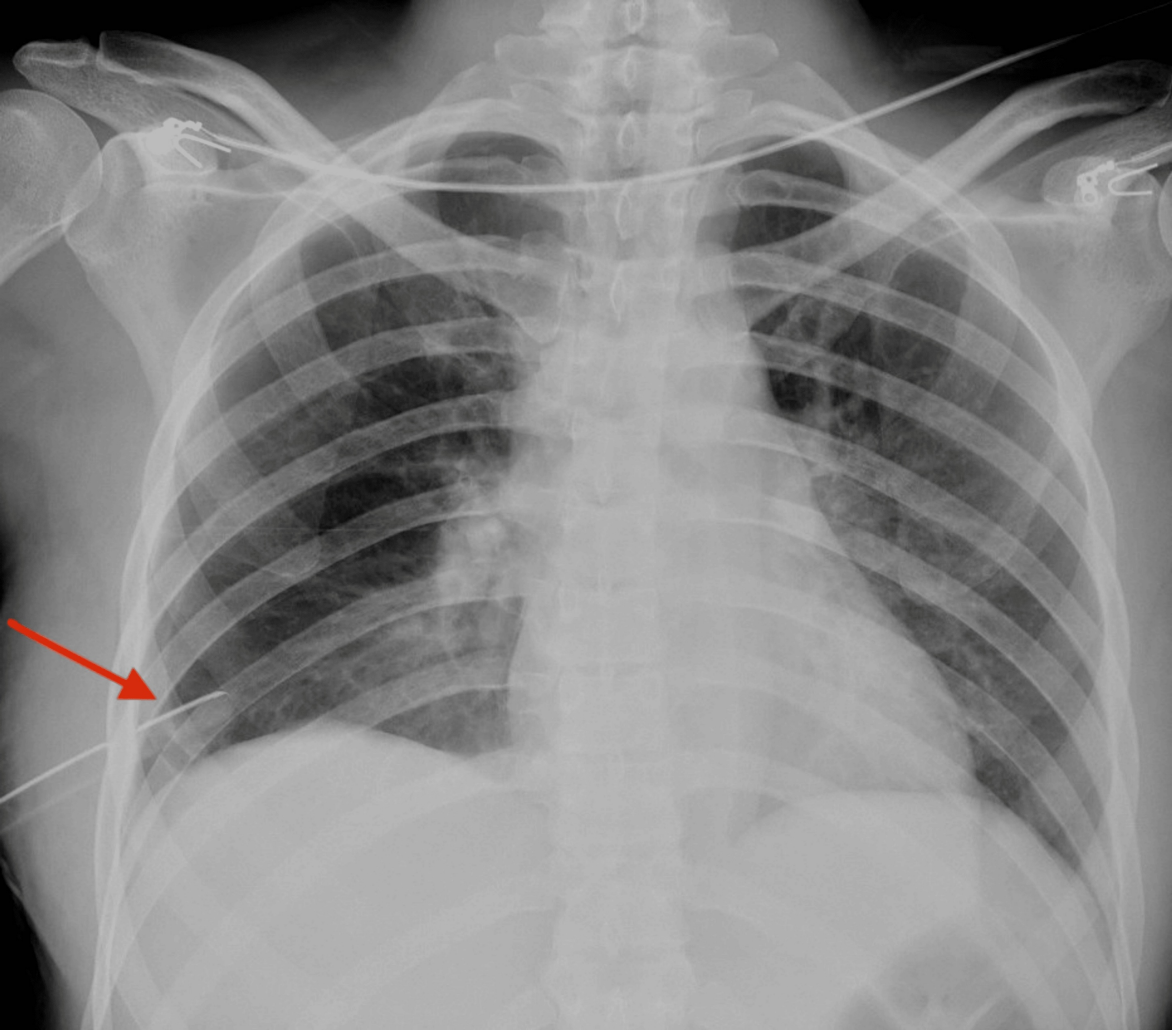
Chest X-ray demonstrating the chest tube The red arrow demonstrates the chest tube in the sixth intercostal space.
